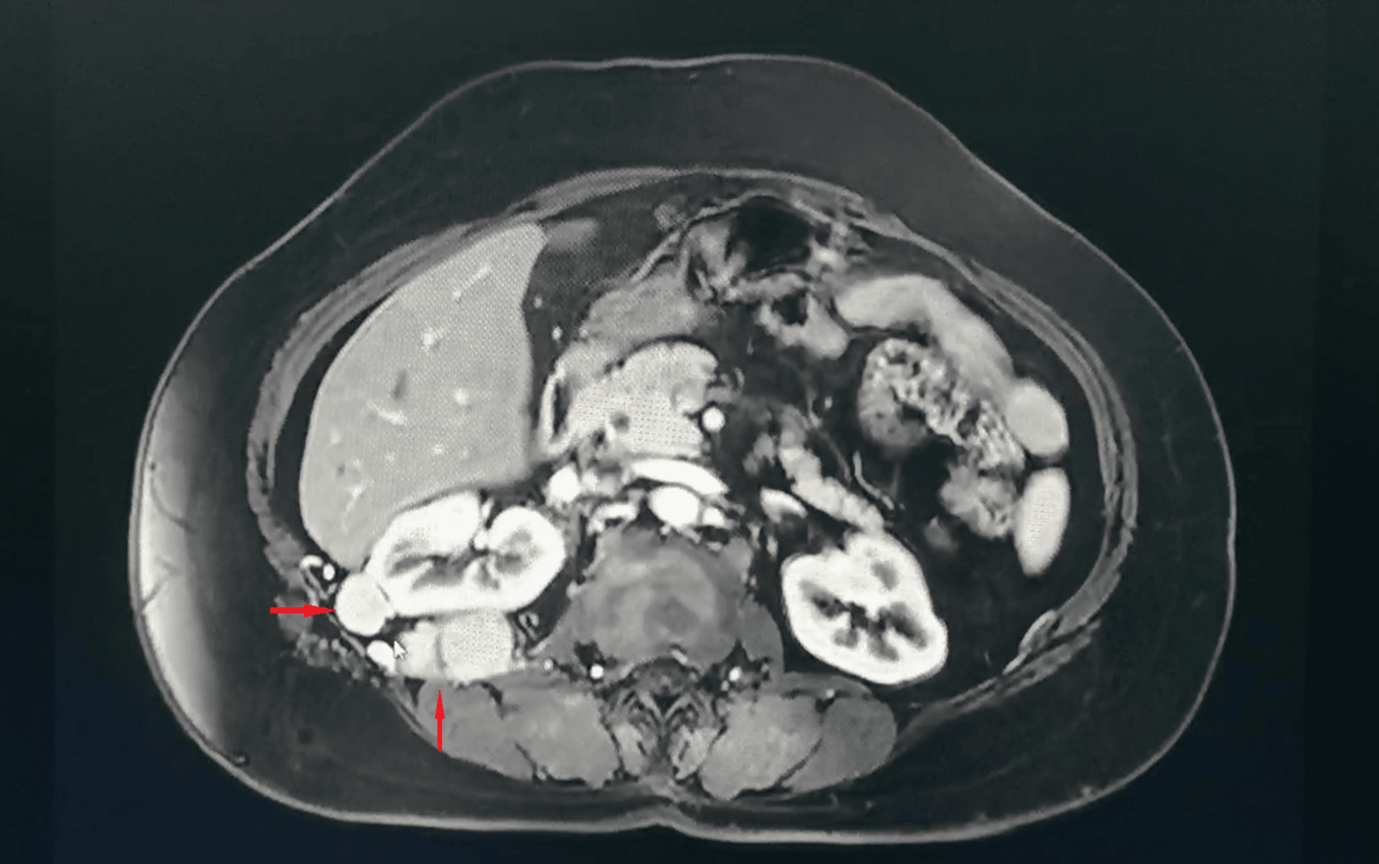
Abdominal MRI findings. The red arrows indicate multiple perinephric masses.
